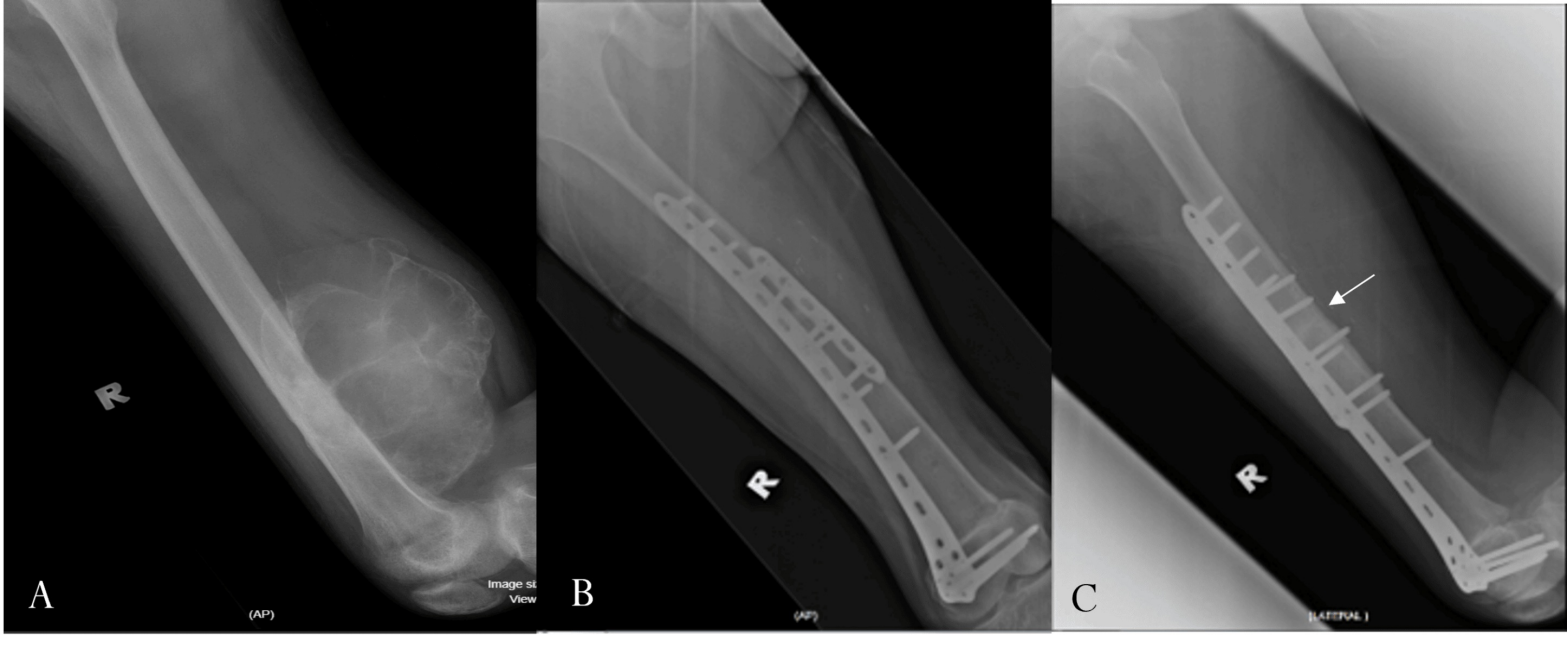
Radiographs of a distal femur osteosarcoma which was resected and reconstructed with intercalary allograft (A) Preoperative lateral view radiograph postadjuvant chemotherapy. (B, C) Anteroposterior and lateral view femur radiographs at one year postsurgery showing radiological union (white arrow)
